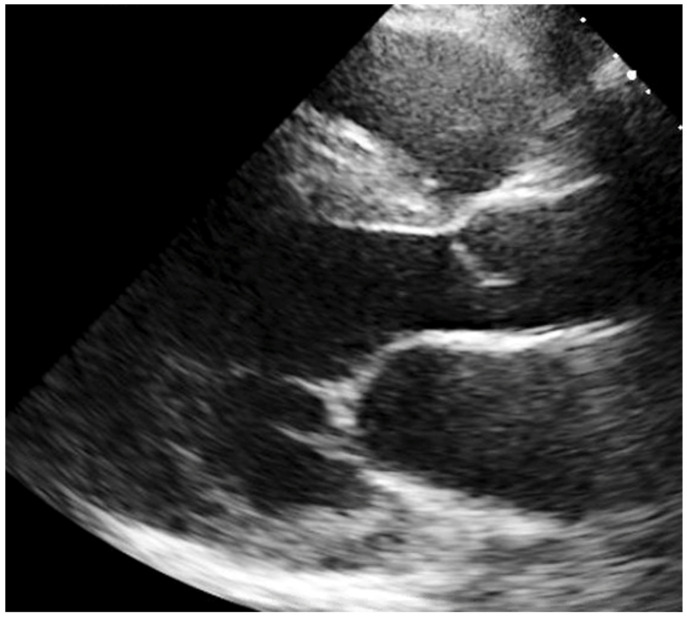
Transthoracic Echocardiogram (TTE) parasternal long axis view demonstrating left ventricular hypertrophy with thinning of the basal inferolateral wall, associated with impaired ventricular function that are typical changes in Fabry disease (FD) cardiomyopathy.
